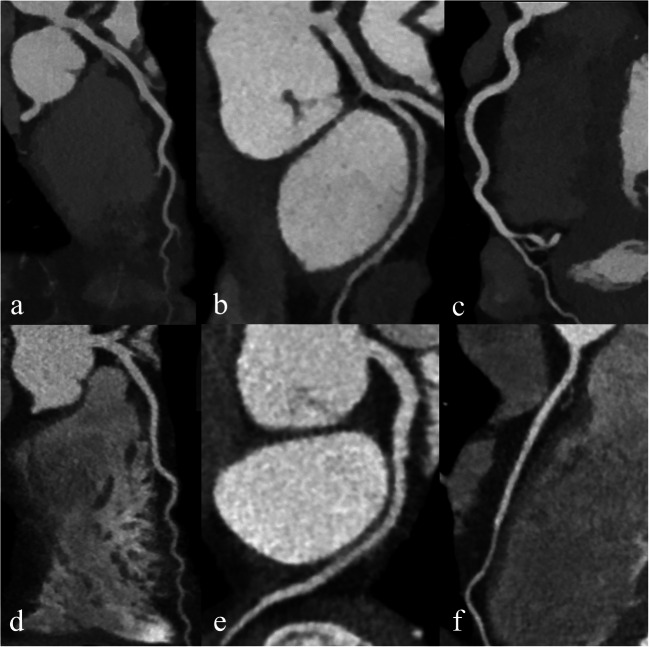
Coronary CT angiography obtained with 40 mL contrast medium injected at 2.5 mL/s and reconstructed as virtual monoenergetic images at 55 keV, depicting the LAD, LCx, and RCA (a–c), in a 68-year-old male. Coronary CT angiography obtained with 70 mL contrast agent injected at 5 mL/s and reconstructed as conventional polychromatic images, depicting the LAD, LCx, and RCA (d–f), in a 48-year-old female. LAD, left anterior descending; Cx, circumflex artery; RCA, right coronary artery; keV, kilo-electronvolt
